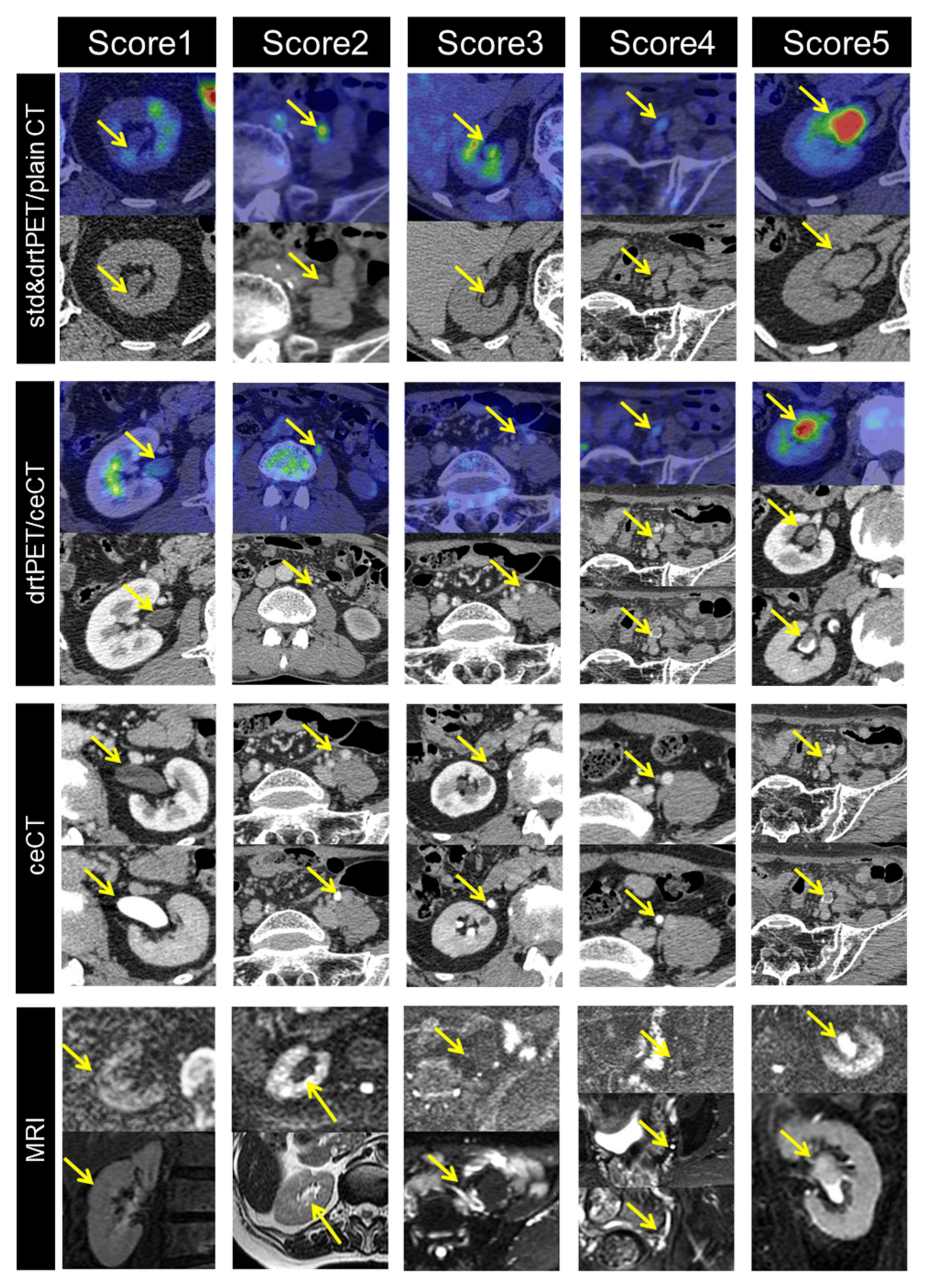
Interpretation criteria of each modality. ceCT: contrast-enhanced computed tomography, stdPET/CT: standard 18F-fluorodeoxyglucose (FDG) positron emission tomography/computed tomography (PET/CT), drtPET/CT: diuretic FDG PET/CT, drtPET/ceCT: diuretic FDG PET/ceCT. The location of the region of interest (ROI) is identified by yellow arrows.
